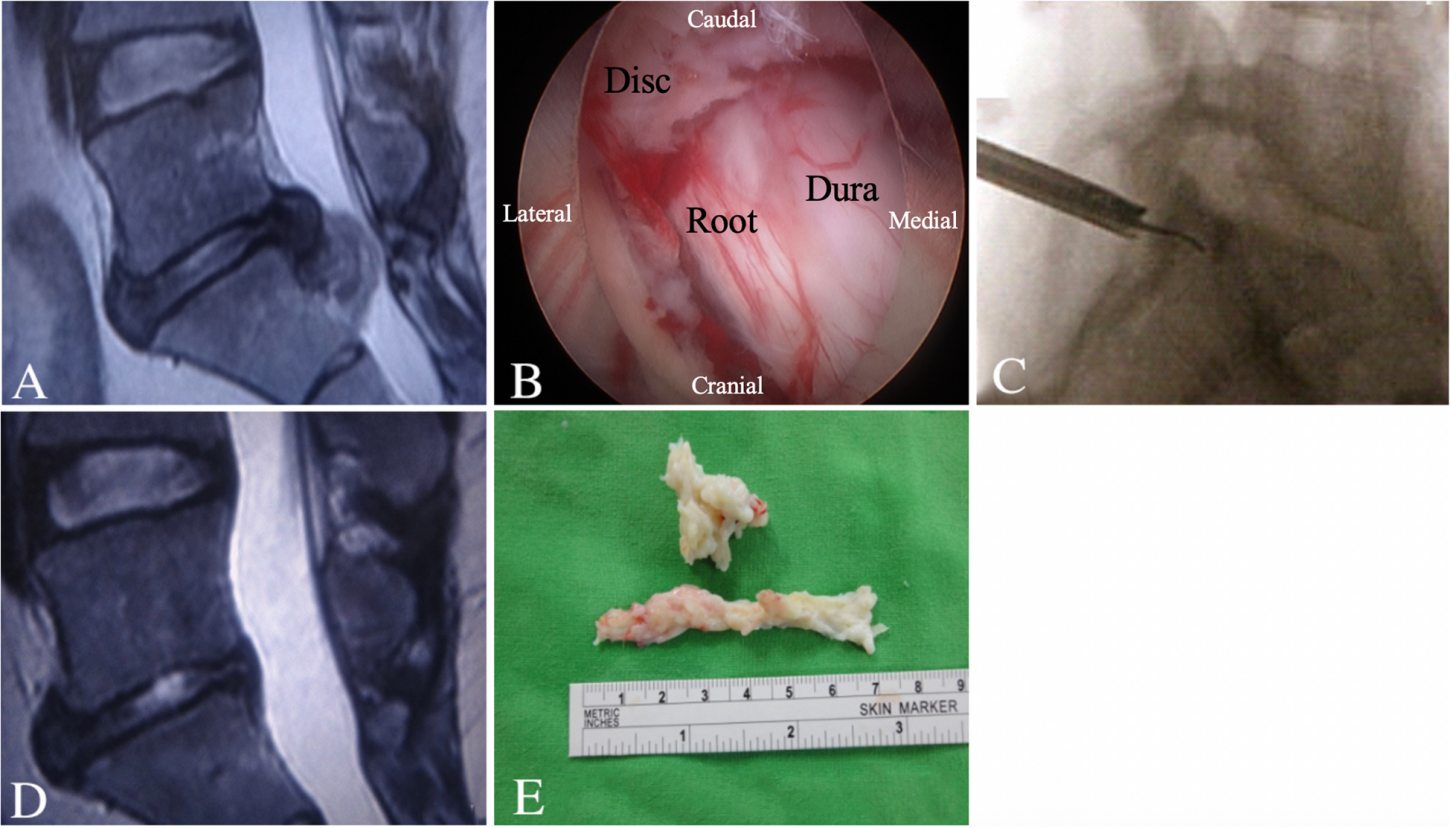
L5–S1 IELD for L5–S1 high-grade downward migration. a Preoperative MRI lateral view showing L5–S1 high-grade downward migration. b Intraoperative endoscopic view of the migrated disc. c Intraoperative fluoroscopic image of the probe at a high-grade migrated disc. d Postoperative MRI lateral view of the removed migrated disc. e Removed disc
